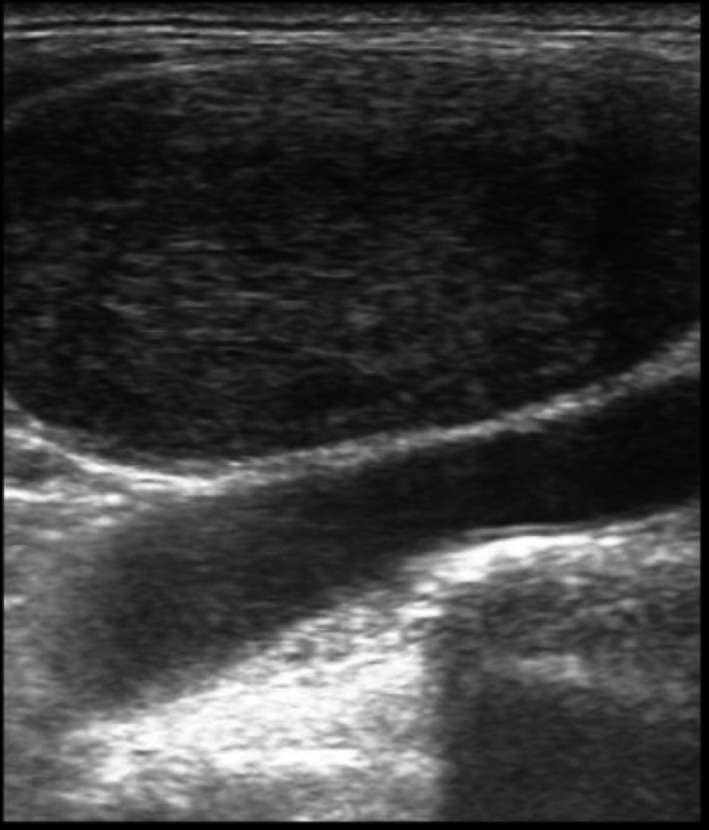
Sonography of the left supraclavicular swelling showing an oval, hypoechoic enlarged lymph node with effaced central hilum. The brachiocephalic vessel and cortex of the left clavicle are seen posteriorly
